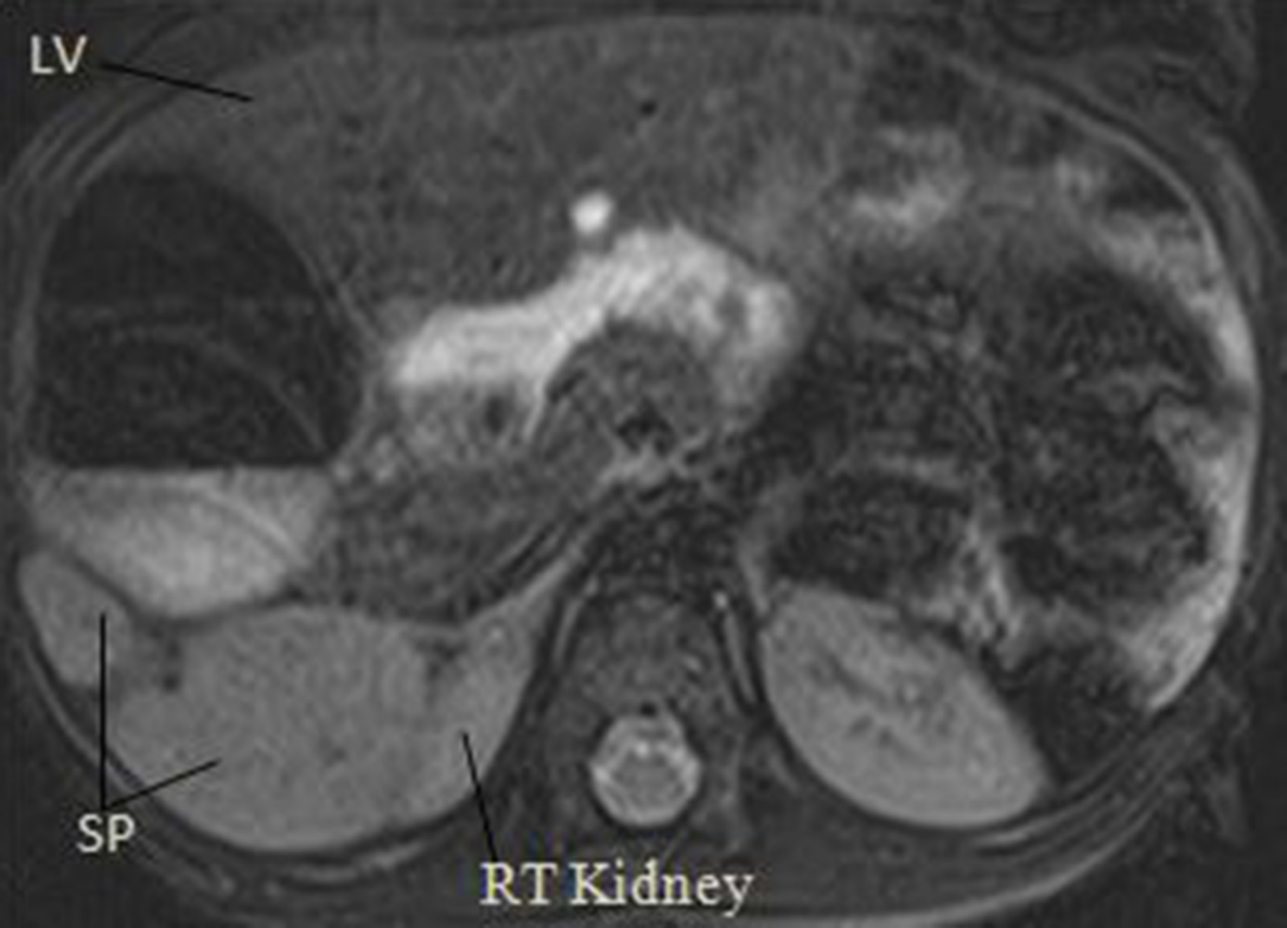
An axial free-breathing abdominal MRI scan showing multiple SPs and the RT. LV, liver; RT, right kidney; SP, spleen.
